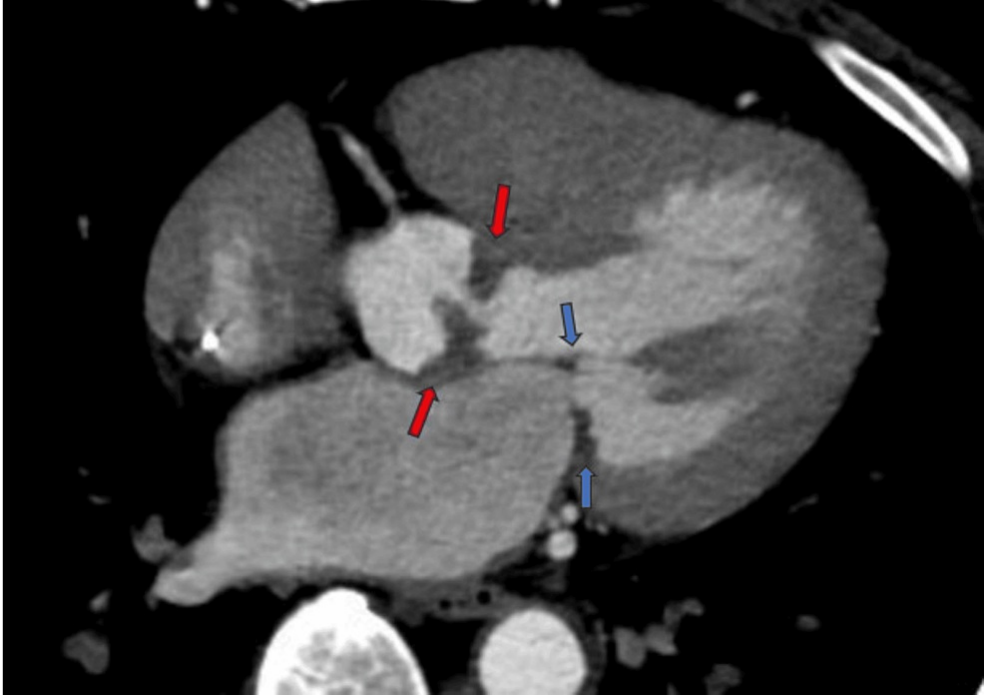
Computed tomography angiogram of the heart showing extensive aortic valve (red arrows) and mitral valve thickening (blue arrows)
